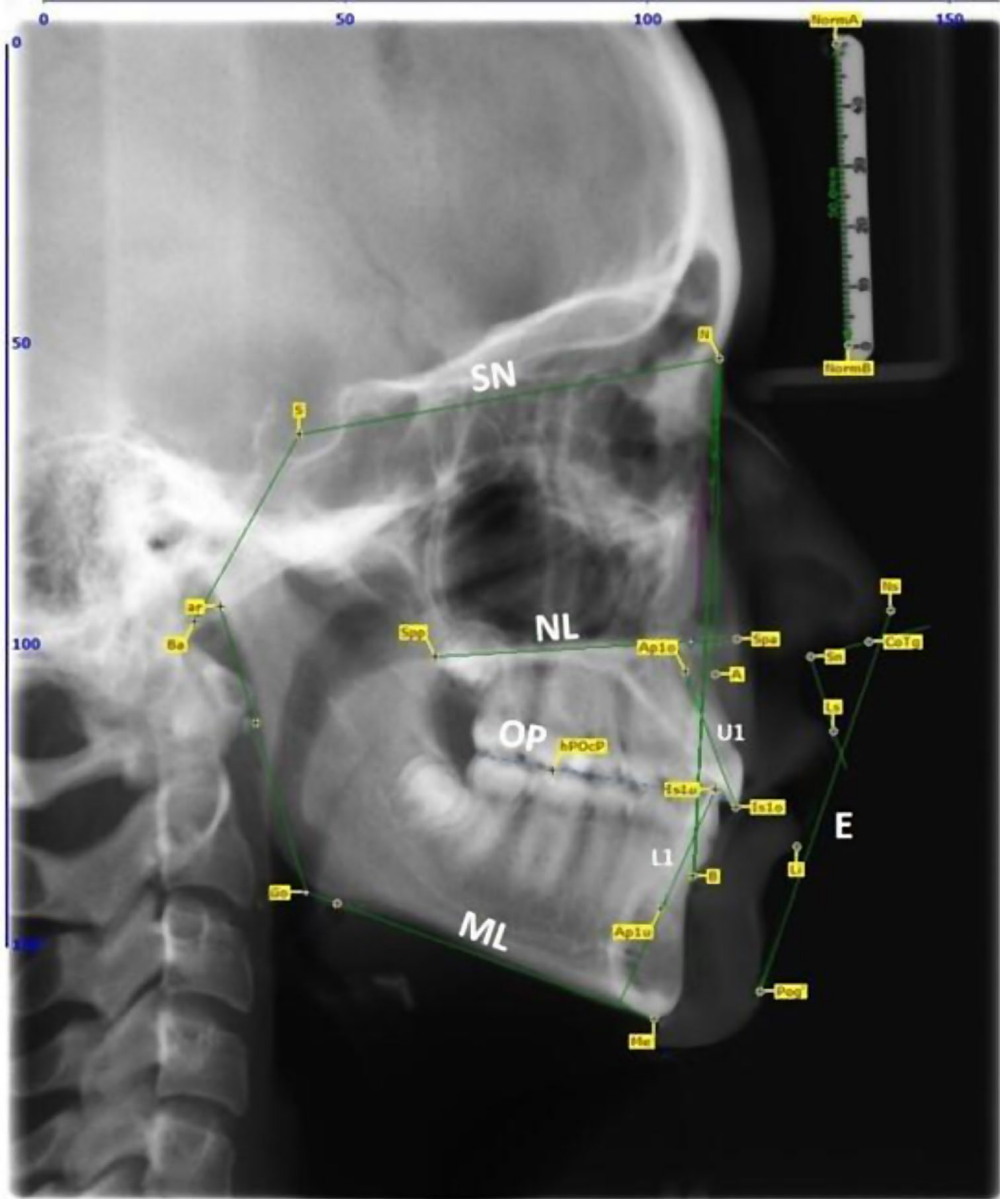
Overview of the landmarks used on the cephalometric radiographs and the linear and angular parameters calculated from them according to Schwarz and Segner and Hasund
